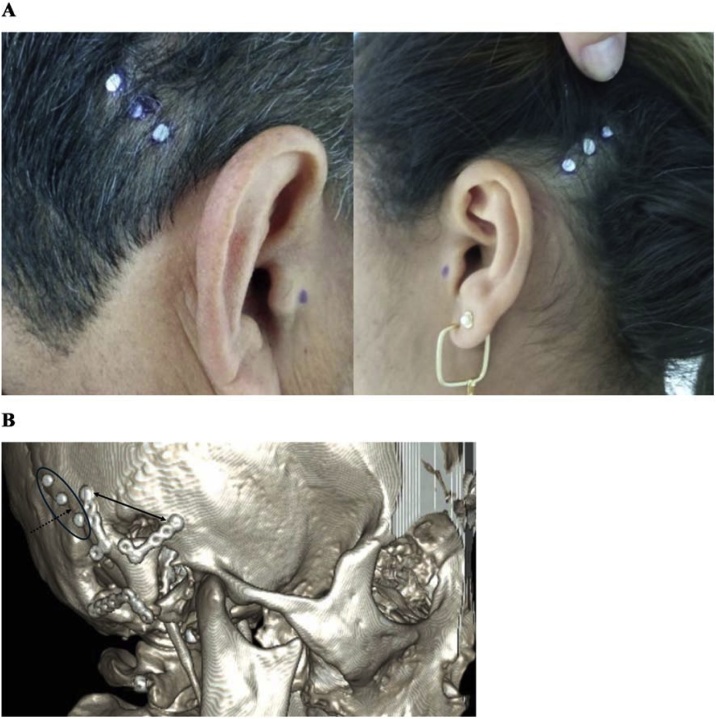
(A) Three optional points for implant placement estimated and marked using Gutta-Percha radiopaque material fixed using a thin layer of elastic collodion. (B) Example of the tridimensional reconstruction of the Computed Tomography (CT) images showing fixation plates (solid arrow) from a previous cranial surgery and the three optional points (circle) marked in a cranial suture line (dotted arrow).
